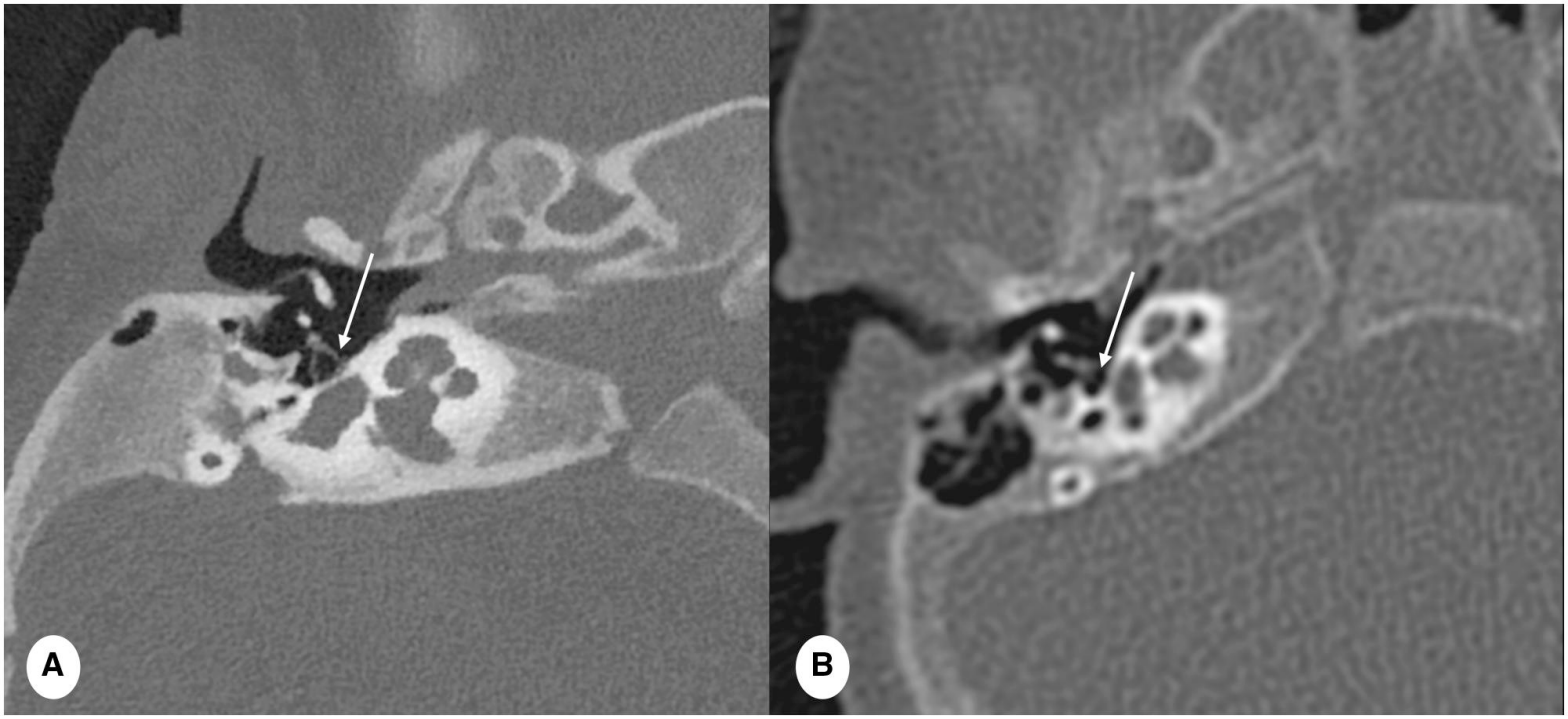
Comparison of temporal bone imaging performed with PCD-CT and EID-CT. PCD-CT scan in infant of 3 months, (A) slice thickness 0.2 mm, reconstruction kernel Hr84 and IR Q3 and EID-CT image in infants of 5 months, (B) slice thickness 0.6 mm, reconstruction kernel Hr56 and IR 3. Note the increase in image sharpness achieved by PCD-CT, clearly delineating the stapes bone (arrow in A) and the contours of the semicircular channels and cochlea. Conversely, EID-CT fails to visualize the stapes bone legs (arrow in B) and shows blurred contours of the internal ear’s structures. Noteworthy, the total dose-length-product for PCD-CT and EID-CT was 56 mGy×cm and 80 mGy×cm, respectively. Remarkably, despite the enhanced spatial resolution of the PDC-CT, these images were obtained at a lower dose compared to standard EID-CT.
